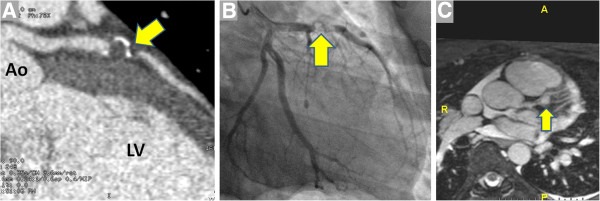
An 11 year-old boy presented to a pediatric hospital with chest and jaw pain while playing one year after a prolonged febrile illness. Initially, CT angiography was performed (A, image courtesy of Dr. Christopher Learn) that showed thrombus in a calcified aneurysm of the left anterior descending coronary artery (LAD, arrow). In the setting of elevation of the serum troponin and possible need for coronary intervention, the patient was transferred to a nearby adult hospital. Invasive angiography (B) showed thrombus nearly occluding LAD that was treated with angioplasty and stent placement. C. Coronary MRA performed in another patient with KD using a navigator-triggered slab prescribed perpendicular to the aortic root demonstrates a 9 mm proximal LAD aneurysm (arrow). LV = left ventricle Ao = aorta.
